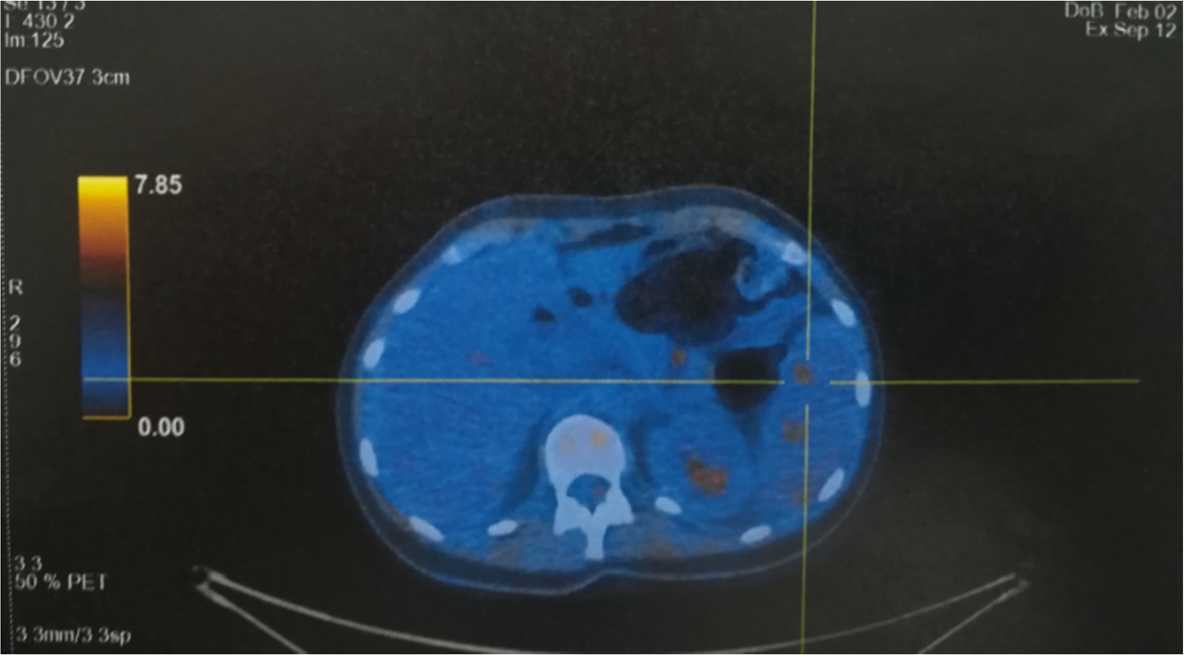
CT/PET Total Body CT/PET Total Body shows hypercaptation of 18F-fluorodeoxyglucose (FDG) in the spleen
